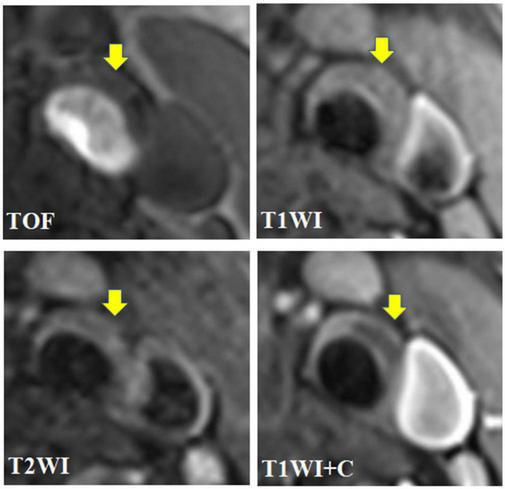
A 67 year-old male presented with intermittent dizziness for 2 weeks. The transverse axial TOF-MRA showed mild stenosis of left common carotid artery and bifurcation with local plaque formation, and the plaque was isointense; HR-MRI axial T1WI showed that the left carotid plaque was isointense; HR-MRI axial T2WI showed low signal in the left carotid plaque and isosignal on the plaque surface; HR-MRI axial T1WI enhancement showed that the surface of left carotid artery plaque showed obvious zonal enhancement, but no enhancement was found in the plaque; To sum up, the plaque components of the left common carotid artery and its bifurcation are the core of lipid necrosis, and the surface fiber cap is intact, which belongs to AHA type V.
